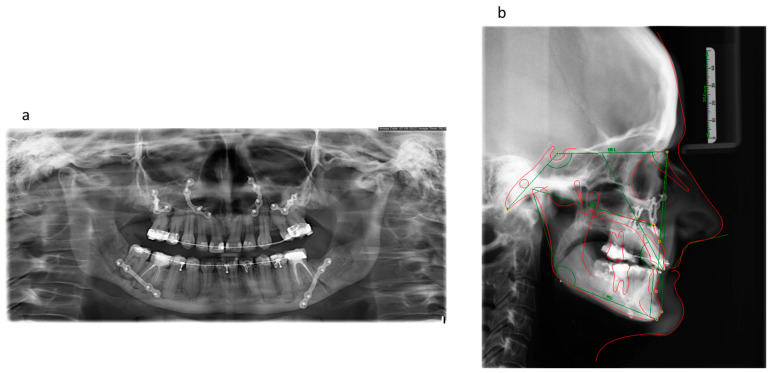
(a) Orthopantomography with orthodontic and surgical intervention and (b) the lateral cephalogram with orthodontic treatment and after surgical intervention: skeletal class I according to ANB (3.4°).
